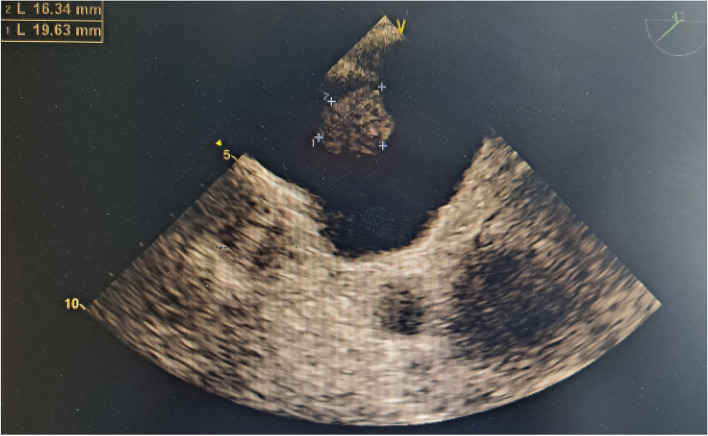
The transesophageal ultrasonography in April 2021. A solid space-occupying lesion in the left atrium at the size of 1.9 cm × 1.6 cm
